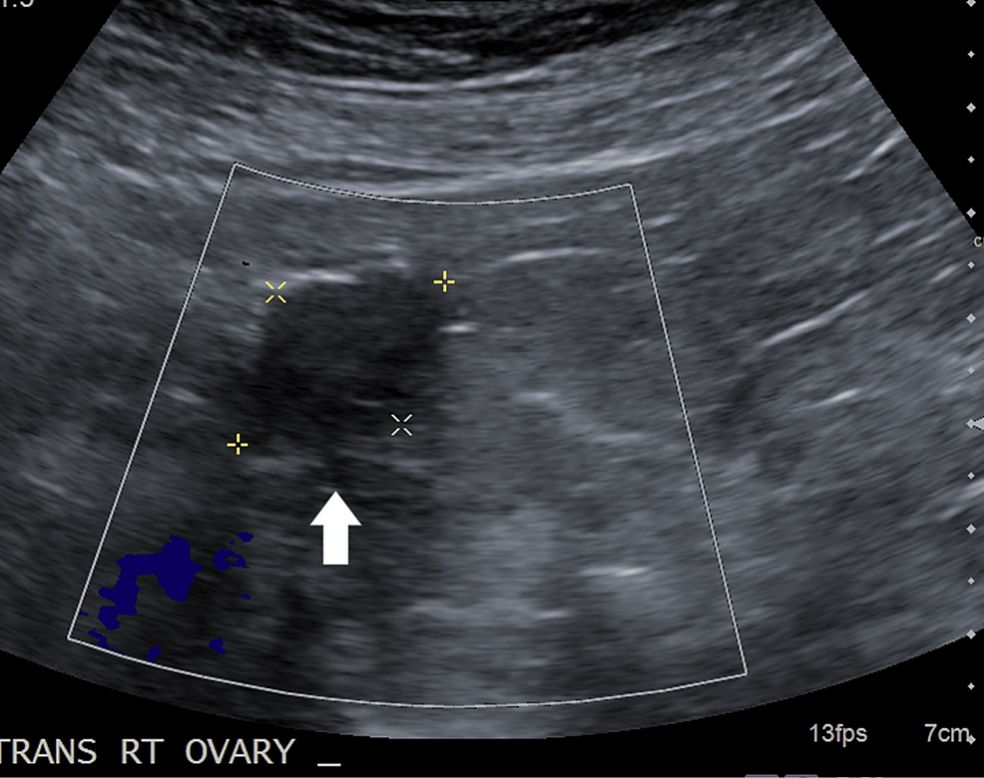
Ultrasound of the right ovary (arrow) A transvaginal ultrasound of the right ovary did not show a tumor. The ovary has a normal size measuring 2.5 × 2.5 × 1.7 cm. The left ovary was not visualized.
